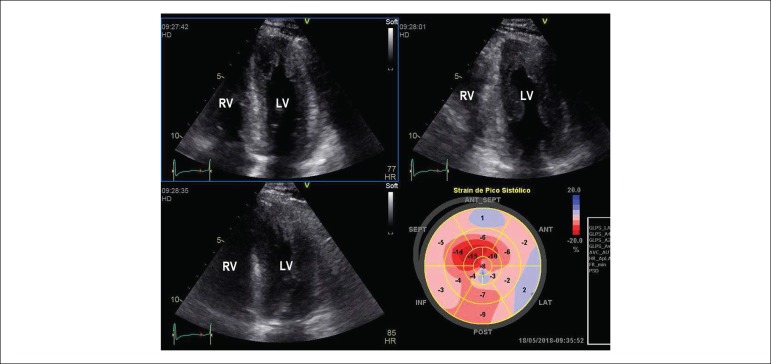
Image obtained from transthoracic echocardiography. 4-chamber apical section showing a diffuse increase in the thickness of the left ventricular myocardial walls. Below, on the right, there is a parametric image of the longitudinal myocardial deformation evaluation by the speckle tracking technique.
